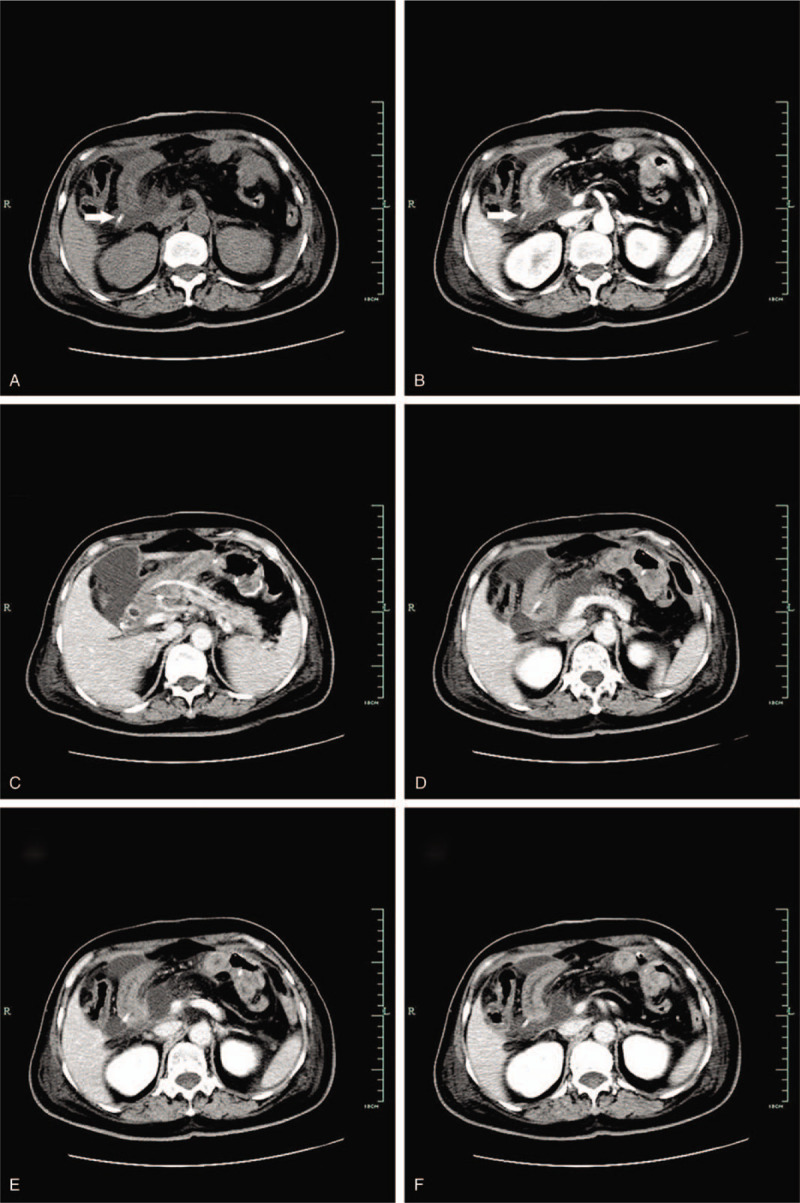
Abdominal enhanced CT 4 days after pancreaticoduodenectomy showed hydrops abdominis, stent (arrow) migration, and bowel limb perforation (A: precontrast CT scan, B: arterial phase, C-F: venous phase). CT = computed tomography.
